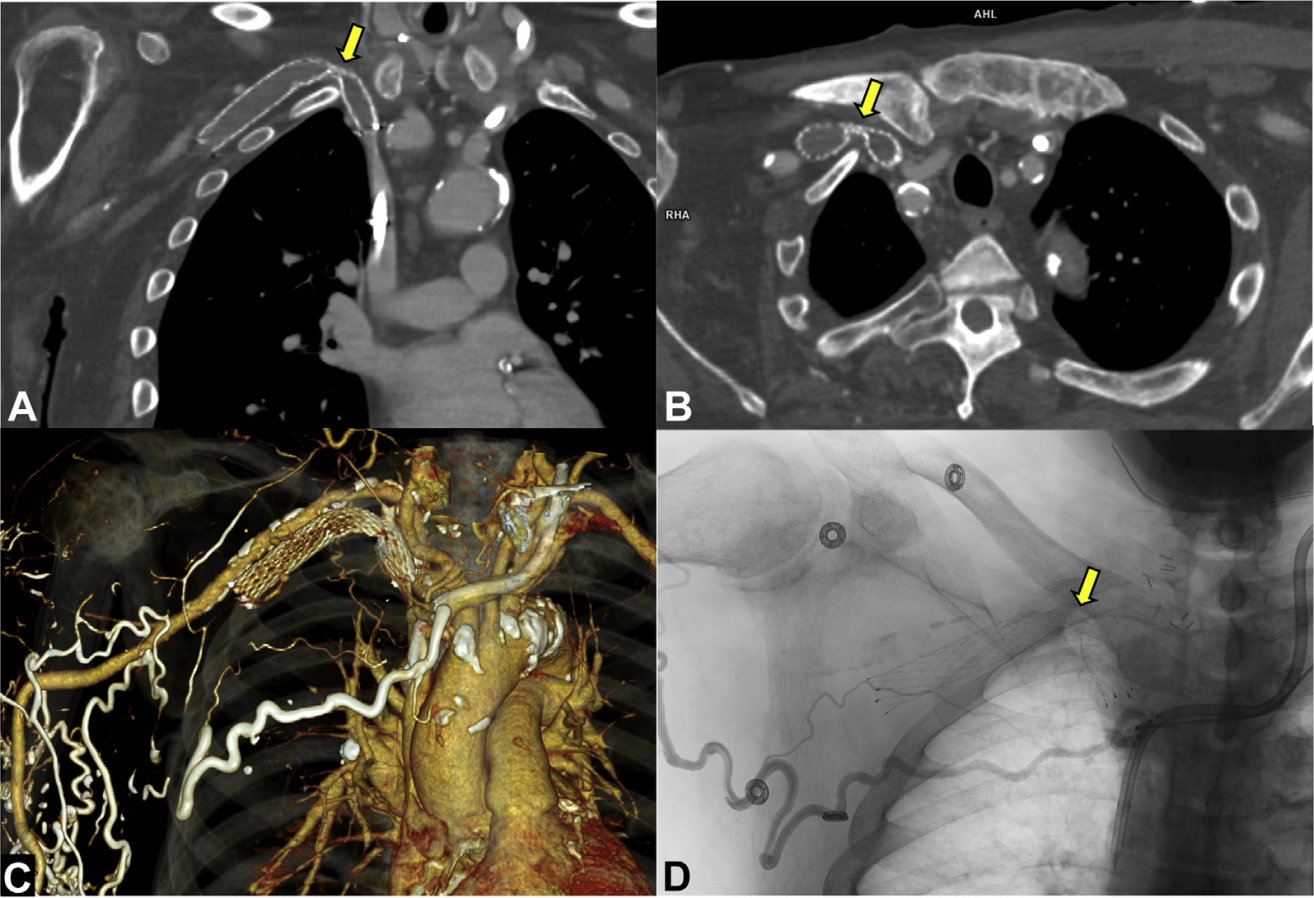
Computed Tomography Venography Demonstrating an Occluded Right Subclavian Vein and Brachiocephalic Vein Stent (A) Coronal, (B) axial, and (C) 3-dimensional volume-rendered reconstruction of computed tomography venography demonstrated no contrast opacification inside the stent. The stent was crushed between the posteromedial end of the clavicle and the first rib (yellow arrows in A and B). (D) Right arm venography showed a deformed, occluded stent (yellow arrow) and collateral vessels.
